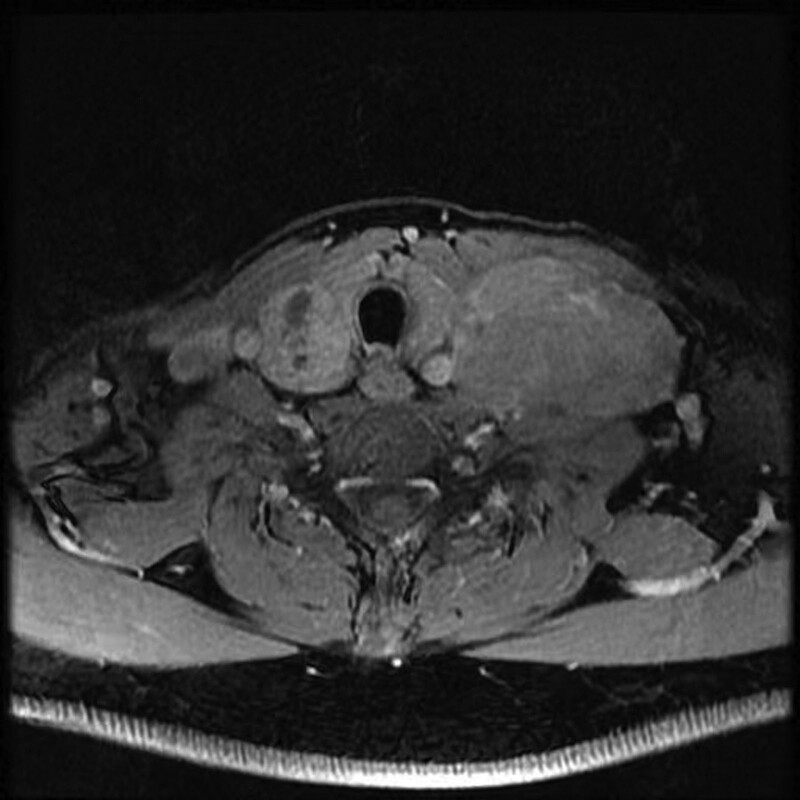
Magnetic resonance imaging revealed a massive abnormal signal shadow in the bilateral para-thyroid areas. Another mass of abnormal signal shadow in the left neck.
